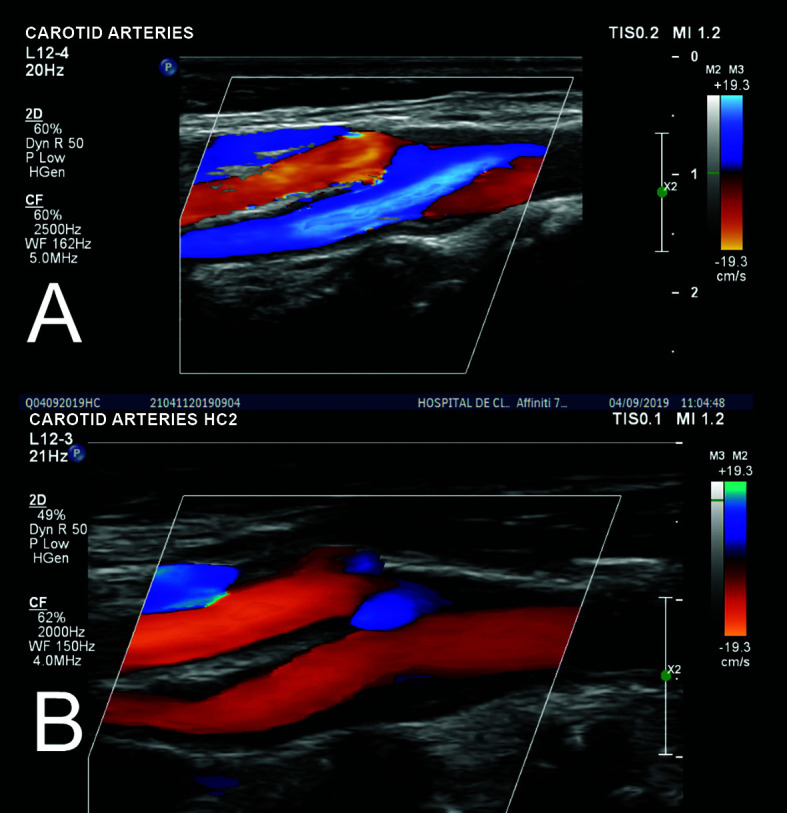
Comparison of the initial (A) and postoperative control (B) Doppler
